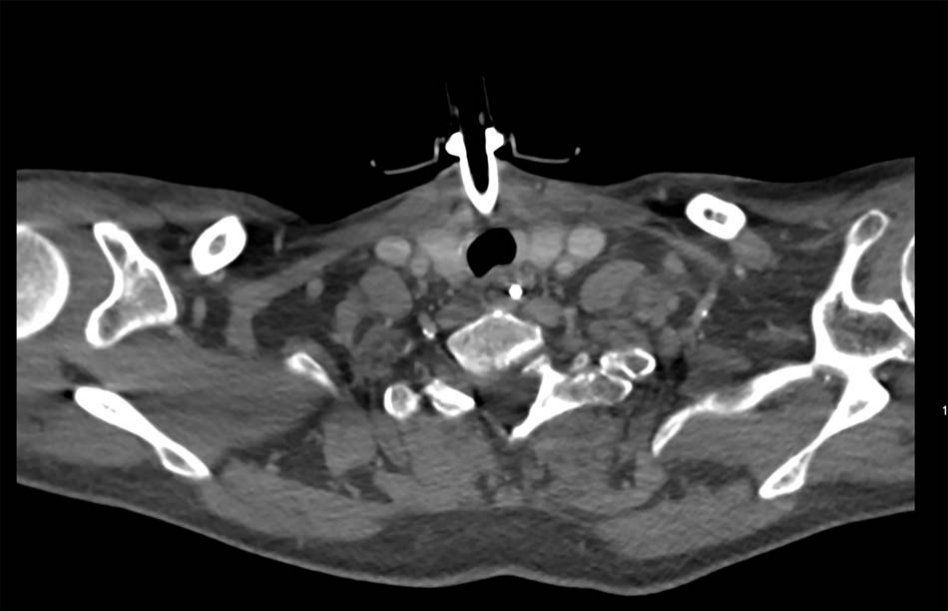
CT showing resolution of the haematoma.
